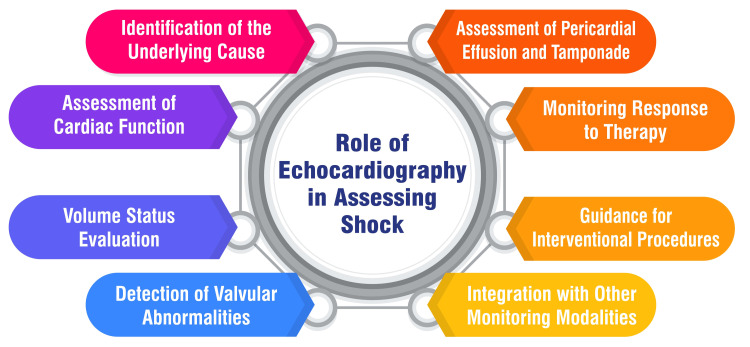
Role of echocardiography in assessing shock The image is created by the corresponding author.
